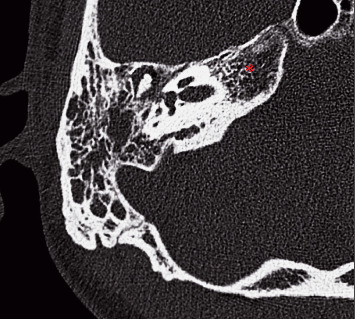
CT temporal bone without contrast in the axial plane with bone windowing. This scan shows mastoid opacification including opacification of air cells in the petrous apex highlighted with the asterisk (^∗^).
